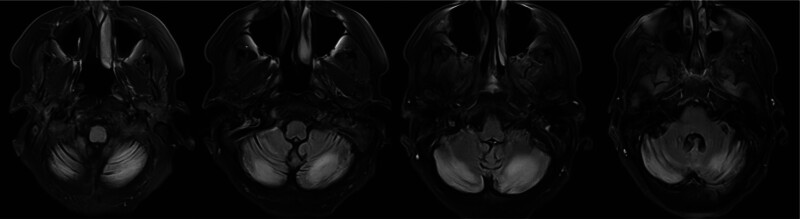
Magnetic resonance imaging of the brain with and without contrast showing an increased T_2_-weighted signal in the bilateral cerebellar hemispheres consistent with central variable PRES. PRES = posterior reversible encephalopathy syndrome.
